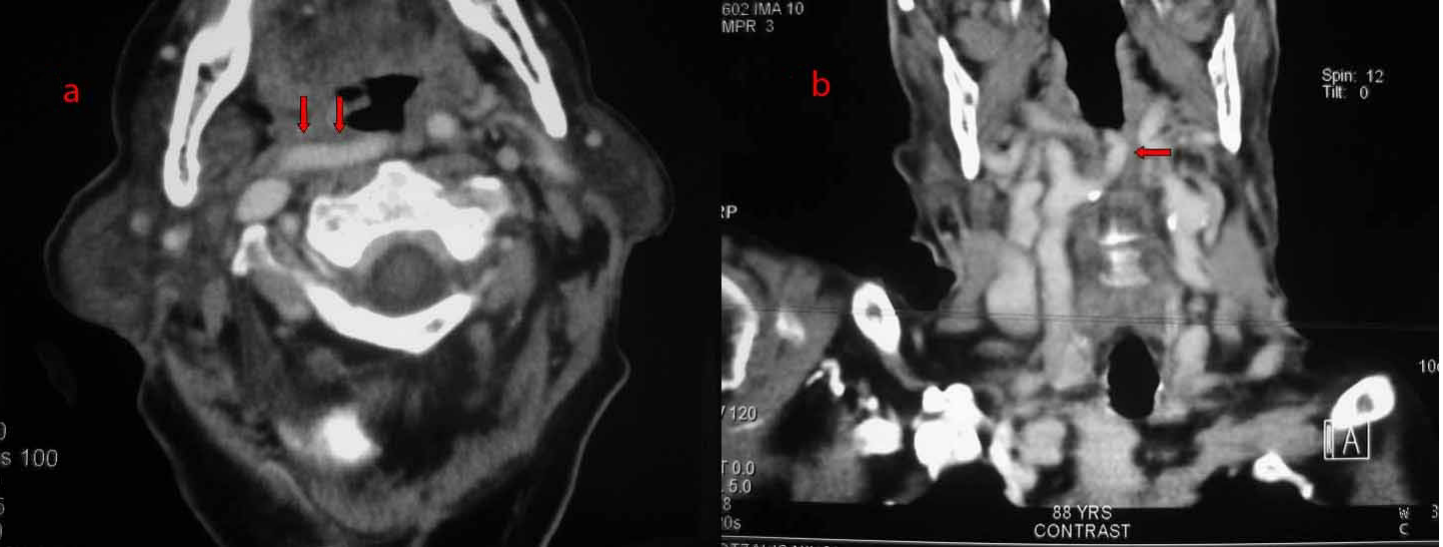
a. CT scan of the neck, following contrast administration. Axial section of the level of the oropharynx, demonstrates the horizontal extension of the right ICA towards the midline and behind the oropharynx. b. Multiplanar reconstruction at the coronal plane demonstrates an angiographic appearance of the vessels of the neck, showing the ectopic portion of the right ICA.
